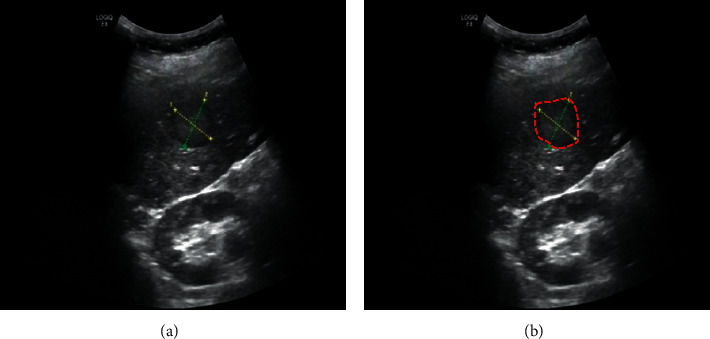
The ultrasound image of breast tumor (a) and the result image of the algorithm for identifying the target area in the ultrasound image (b) (the red curve showed the size of the lesion range).
